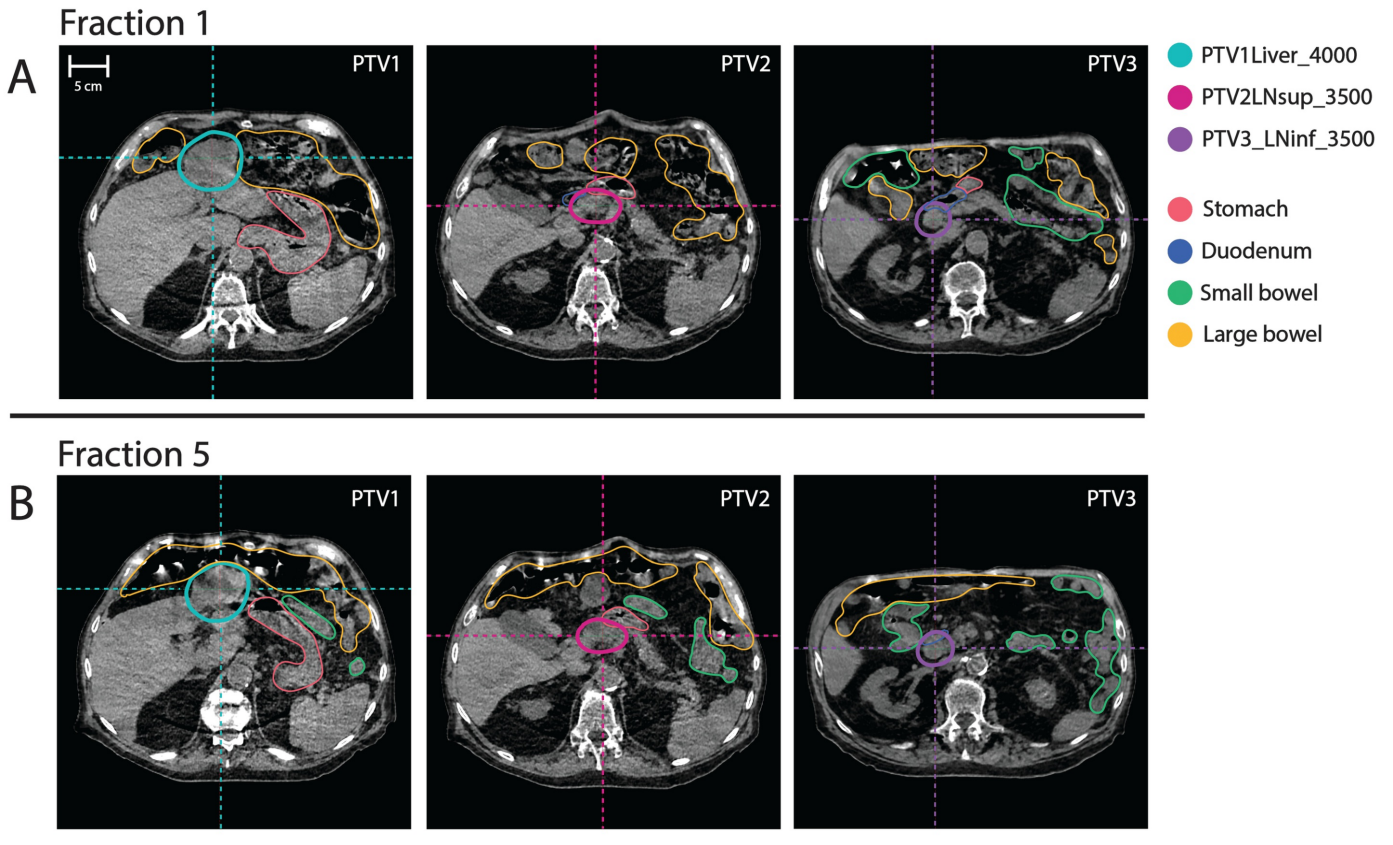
Daily CBCTs for online adaptation Examples of target definitions on fractions 1 and 5 relative to adjacent gastrointestinal (GI) organs at risk (OARs) on cone beam computed tomography (CBCTs) acquired for those fractions.
